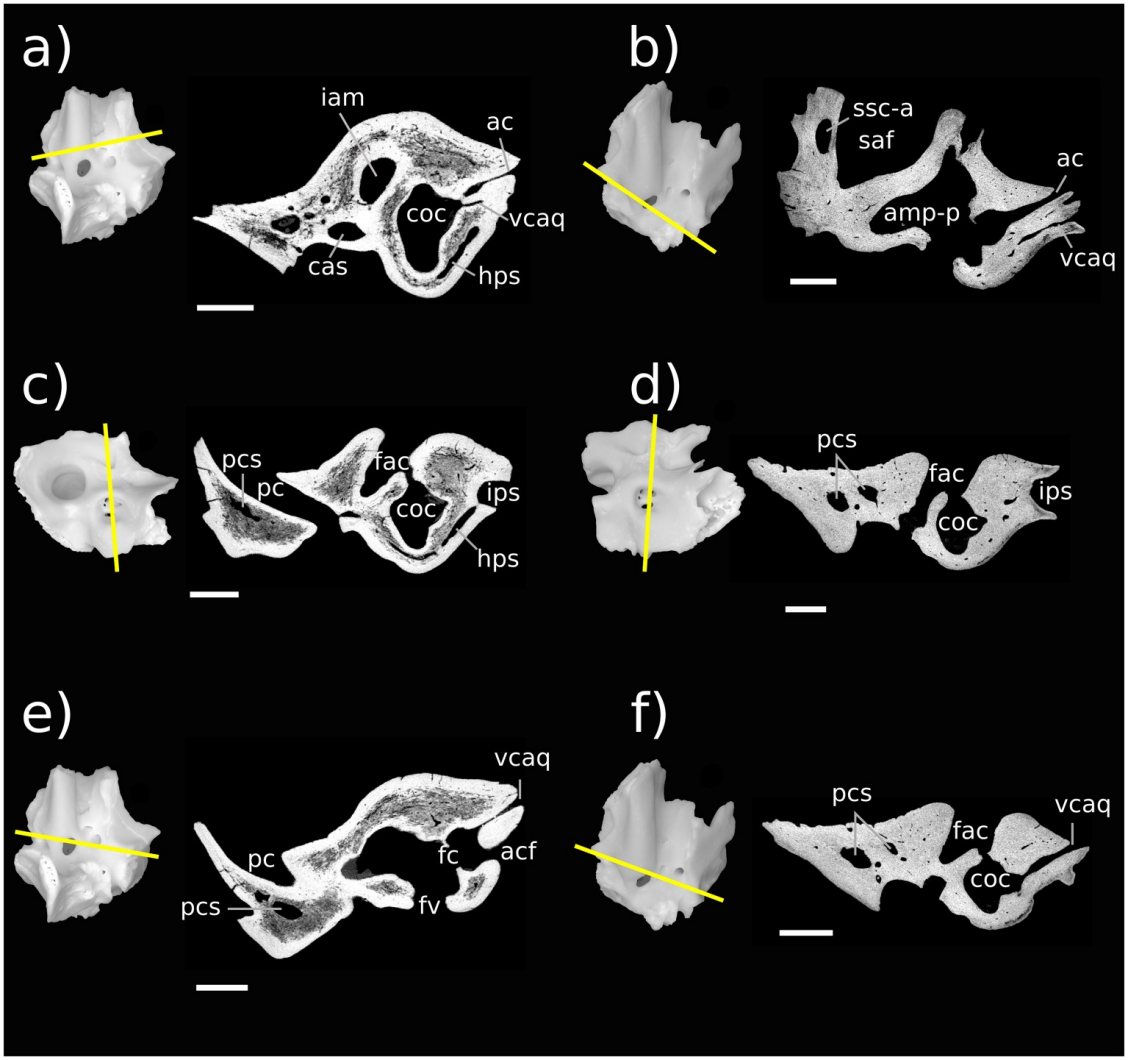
Resliced CT images of Höövör petrosals. a, c, e Höövör petrosal 1 (volume renderings are right-to-left reflected); b, d, f Höövör petrosal 2. a, b are oblique planes through both the cochlear aqueduct and canal for the vein of the cochlear aqueduct; c, d, e, f are coronal planes through the promontorium. Parts c, d are taken from a more posterior plane than e and f to show the hypocochlear sinus in H1. In all images left is lateral and dorsal is approximately toward the top of the page; all scale bars are 1 mm. Refer to list of abbreviations in text for other abbreviations.
